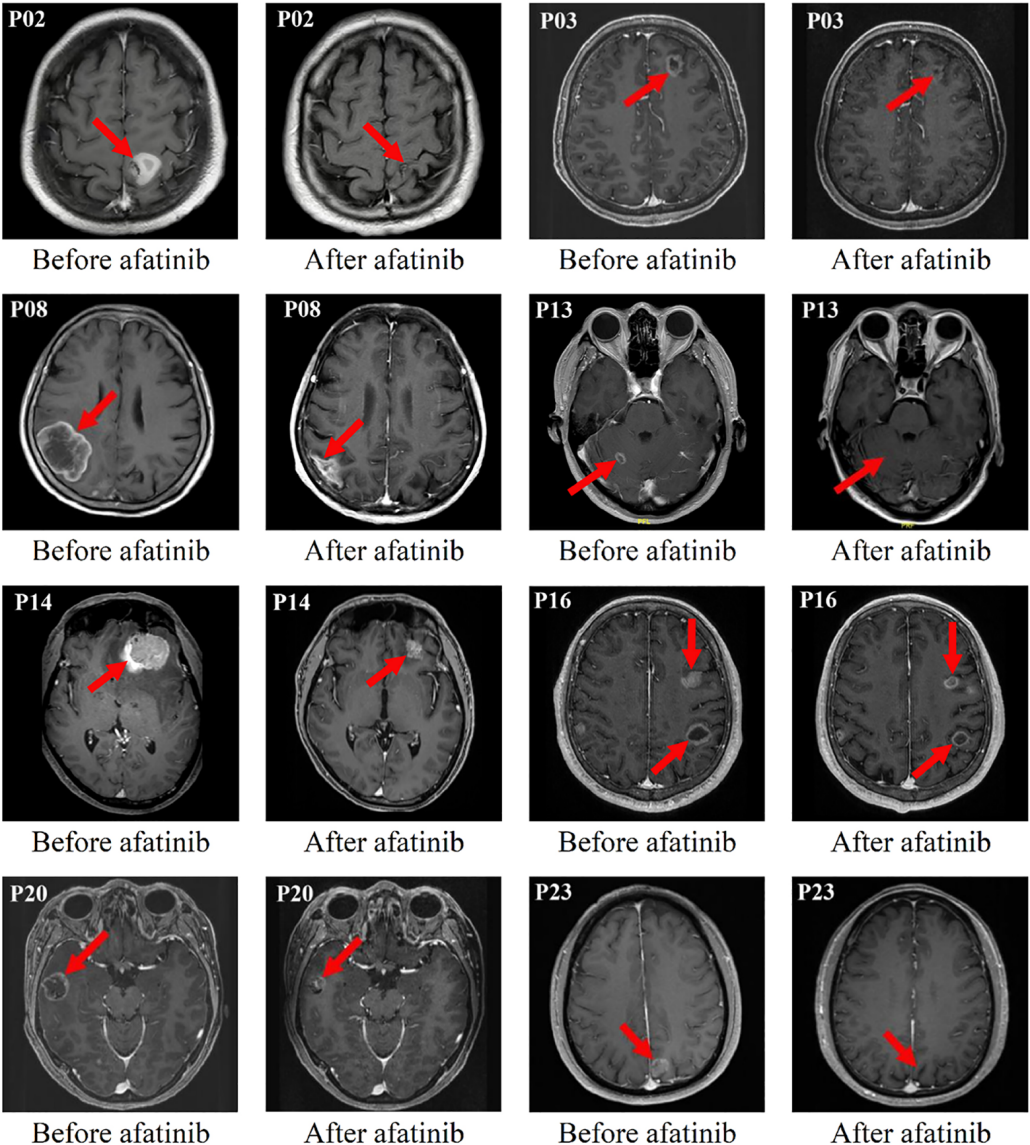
Eight typical examples of brain contrast MRI radiological changes in patients with measurable brain lesions (i.e. The red arrow points).
